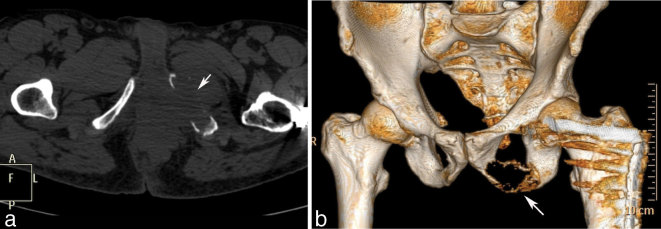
Post-operative axial (a) and volume-rendered (b) CT images showing a lytic lesion of the left inferior pubic ramus (arrows).
